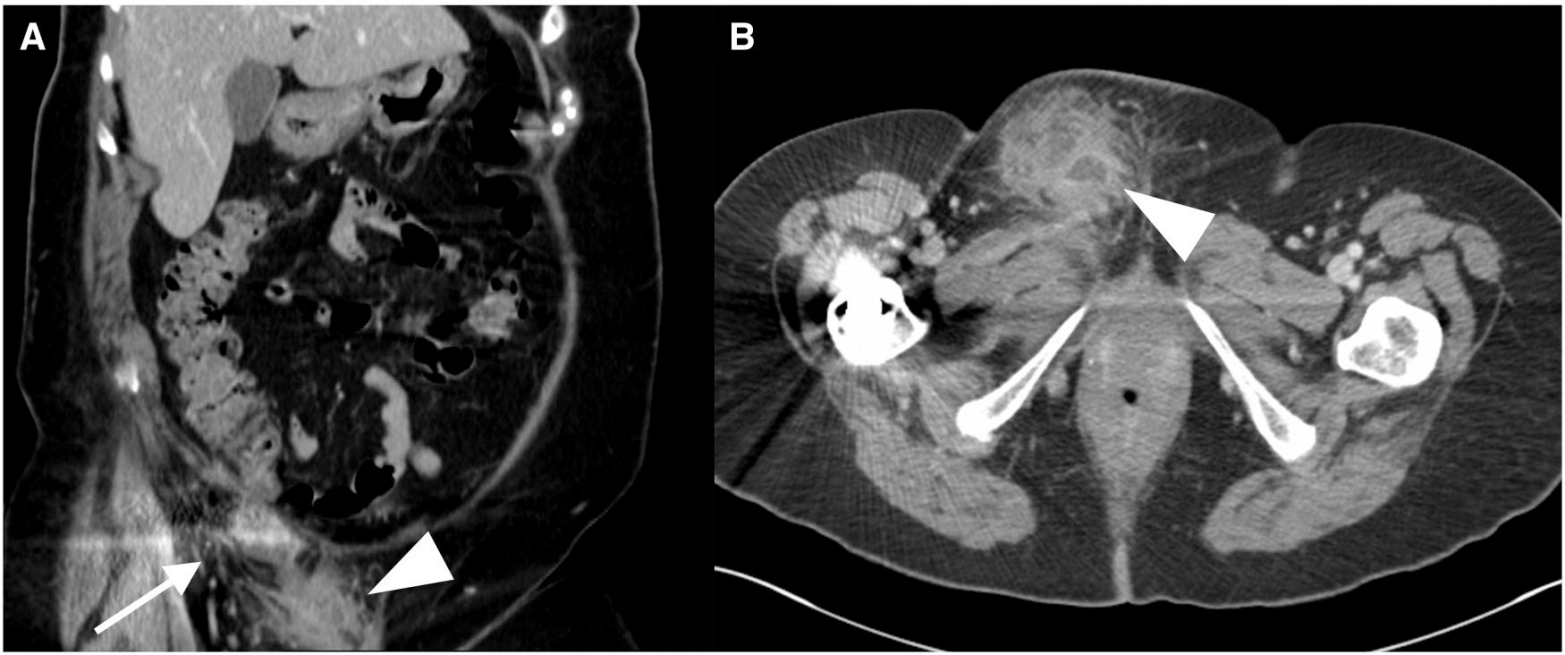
Contrast enhanced CT in the portal phase in coronal (A) and axial (B) planes demonstrating the right inguinal hernia containing the perforated dilated appendix (arrow) with an adjacent abscess tracking to the right labia majora (arrowhead).
